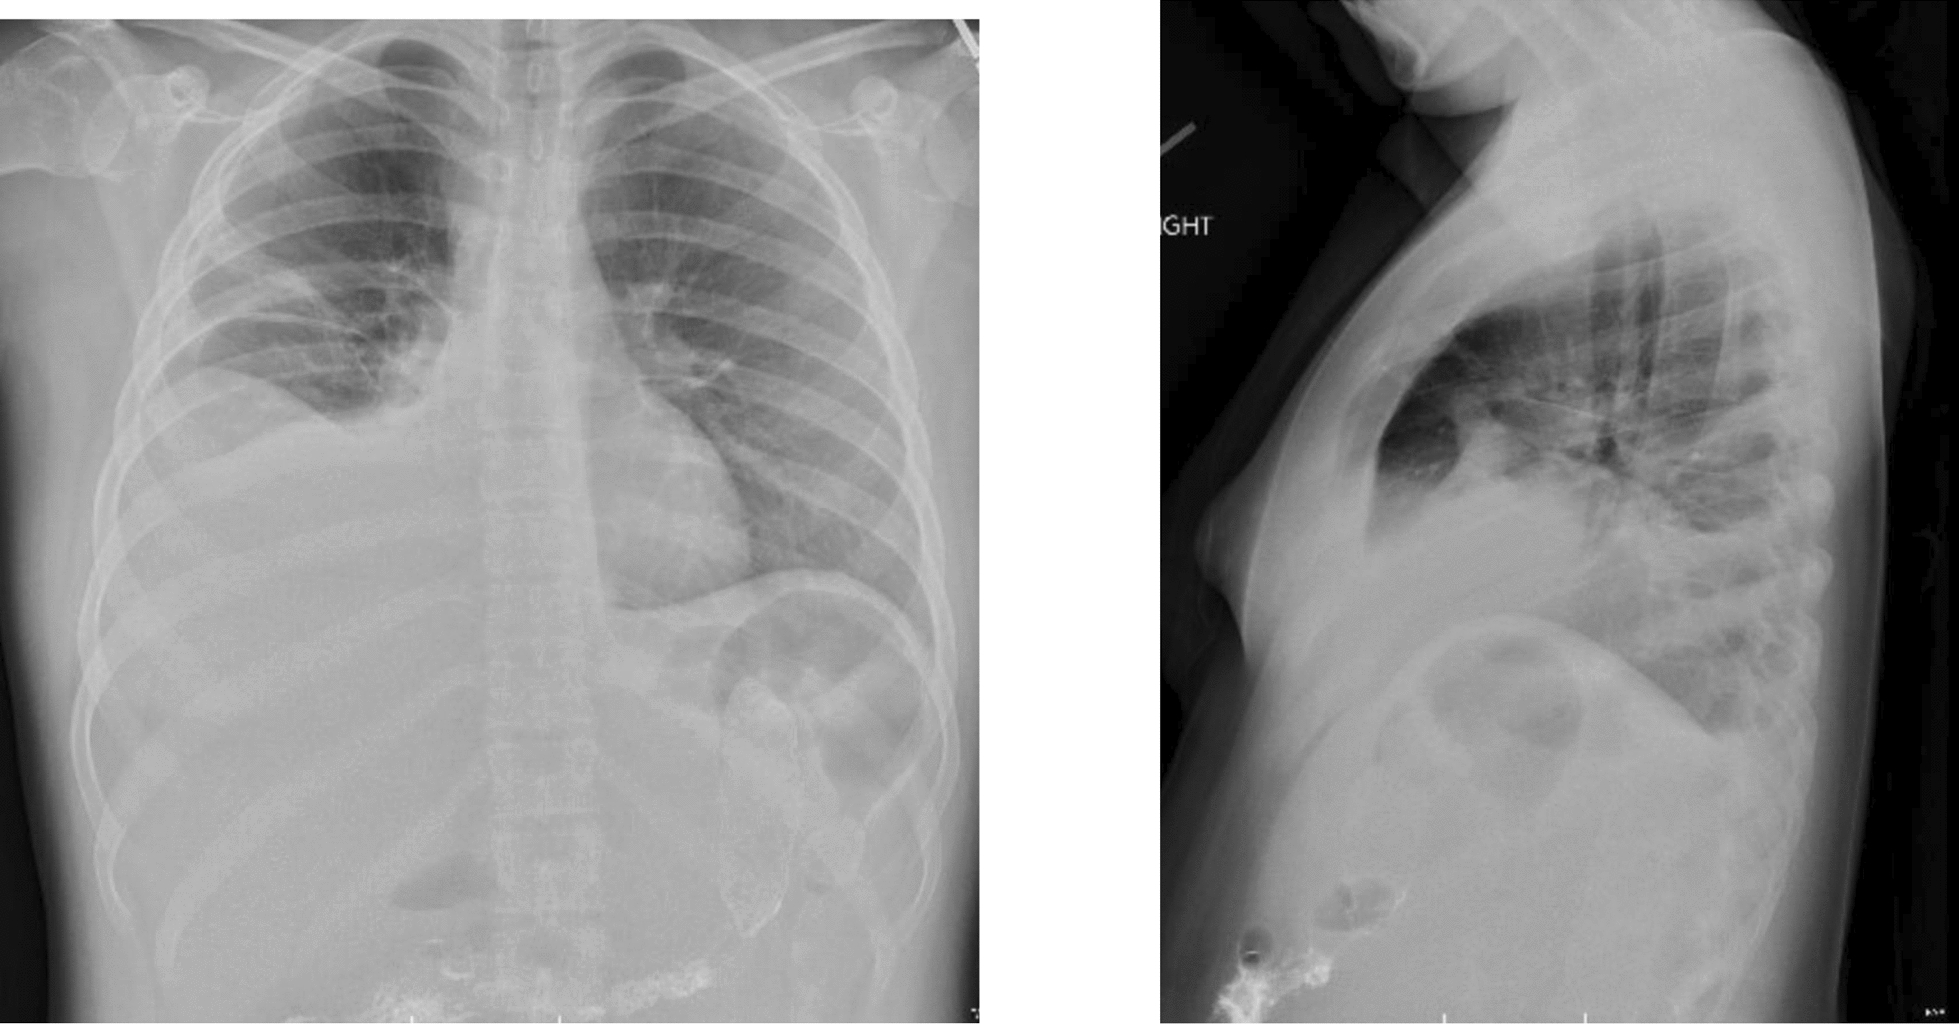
Two-view chest radiograph depicting bilateral pleural effusions (right greater than left) during patient’s first hospitalization
